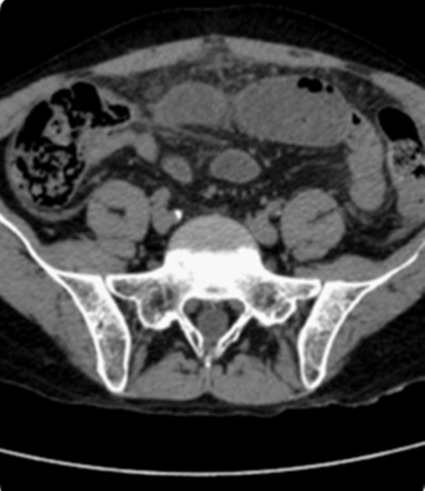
CT scan showing bowel thickening
